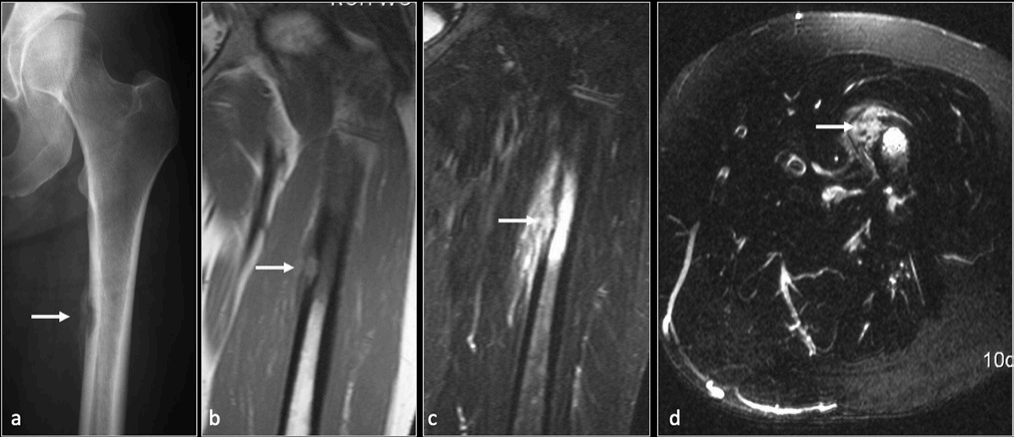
Osteomyelitis with cortical abscess femur. Anteroposterior (a) radiograph of the proximal femur showing lucent lesion in the medial cortex (arrow) with adjacent periosteal reaction. Coronal T1(b), coronal and axial STIR (c, d) MRI images demonstrating intracortical abscess (arrow) with extensive marrow and adjacent soft tissue oedema. STIR, short tau inversion recovery.
